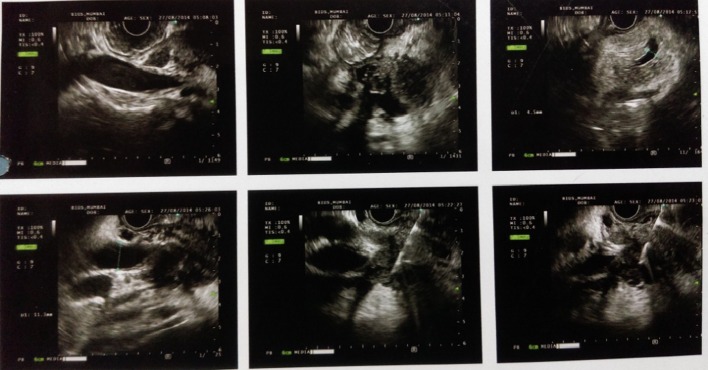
Endoscopic ultrasound (EUS) showing hypoechoic lesion in pancreas head causing dilation of CBD (11.3 mm) and PD (5.3 mm) with compression of portal vein and multiple peripancreatic and perilesional nodes present.
